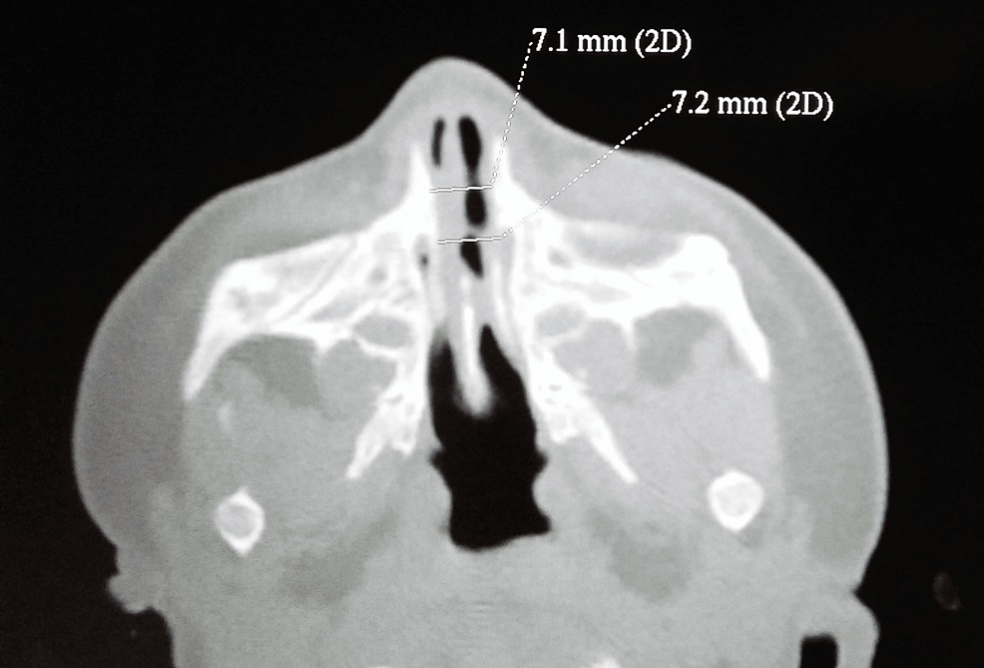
Postoperative CT scan Six weeks postoperative CT scan showing nasal aperture width of 7.1 mm.
